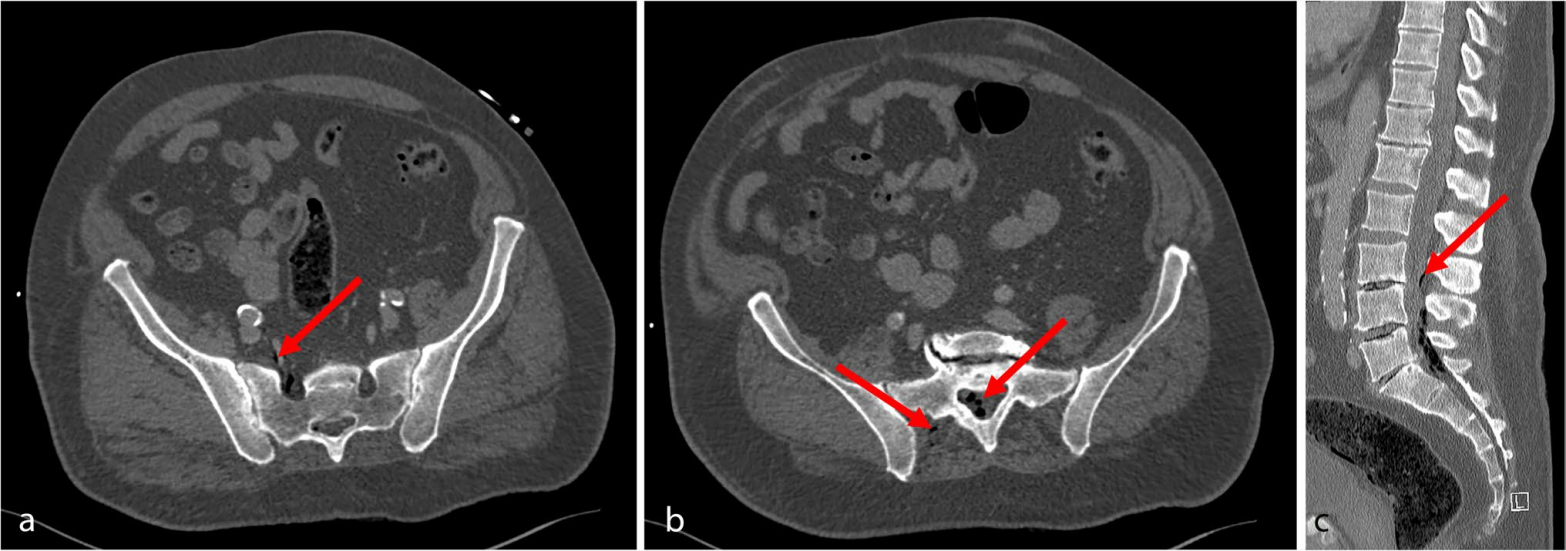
Computed tomography (CT) imaging 2 h after petroleum exposition. Axial (a and b) and sagittal (c) imaging reveal intramuscular gas distribution (red arrows), along the nerve roots (a) and, likely, between the cauda equina fibers (b) and ascendence up to the level of L3 (c), suggestive for intrathecal diffusion. Due to obesity of the patient, primary intrathecal injection was considered unlikely
